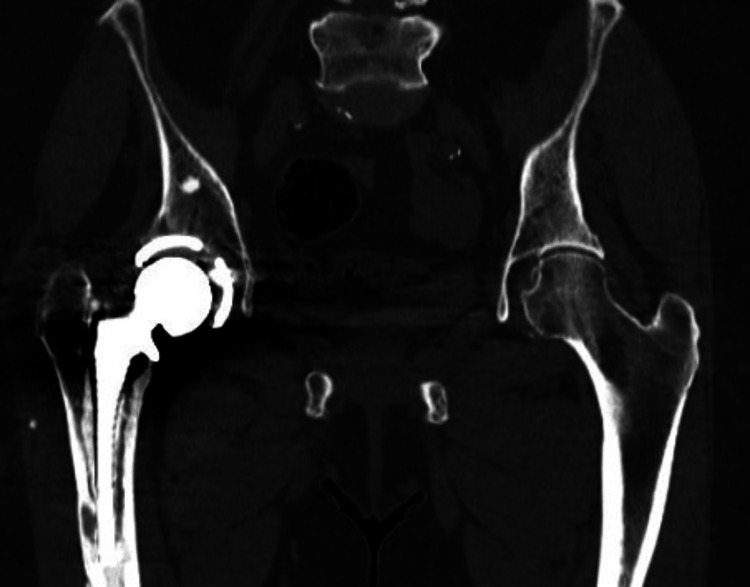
Pelvic non-contrast CT scan in coronal view. Hypodense zones between cement mantle and bone in zones 2, 3, 5, 6, and 7 of Gruen, and hypodensity in the superolateral third of the stem and the cement mantle.
